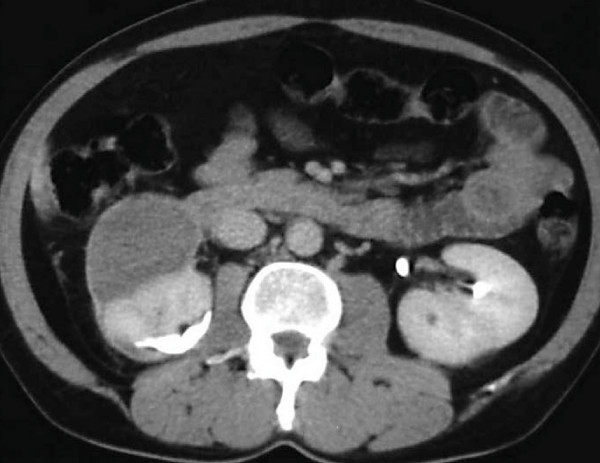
The six-month follow-up computed tomography scan shows total elimination of the left subcapsular hematoma and a significant reduction of the retroperitoneal hematoma on the right.
